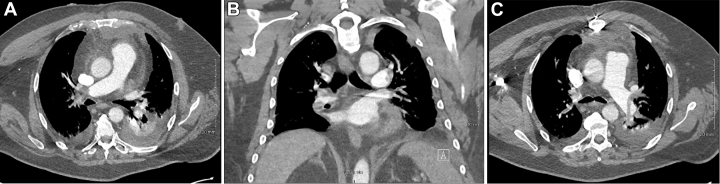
Chest Computed Tomography Angiography Demonstrating Bilateral Pulmonary Emboli (A) Contrast-enhanced imaging shows a linear intraluminal filling defect within several right upper lobe segmental and subsegmental pulmonary arteries. (B) A large filling defect is present in the right interlobar pulmonary artery with extension into the right middle and lower lobe branches. (C) Axial contrast-enhanced imaging demonstrates a linear intralinal filling defect within the left interlobar pulmonary artery extending into the left upper and lower lobe segmental branches.
